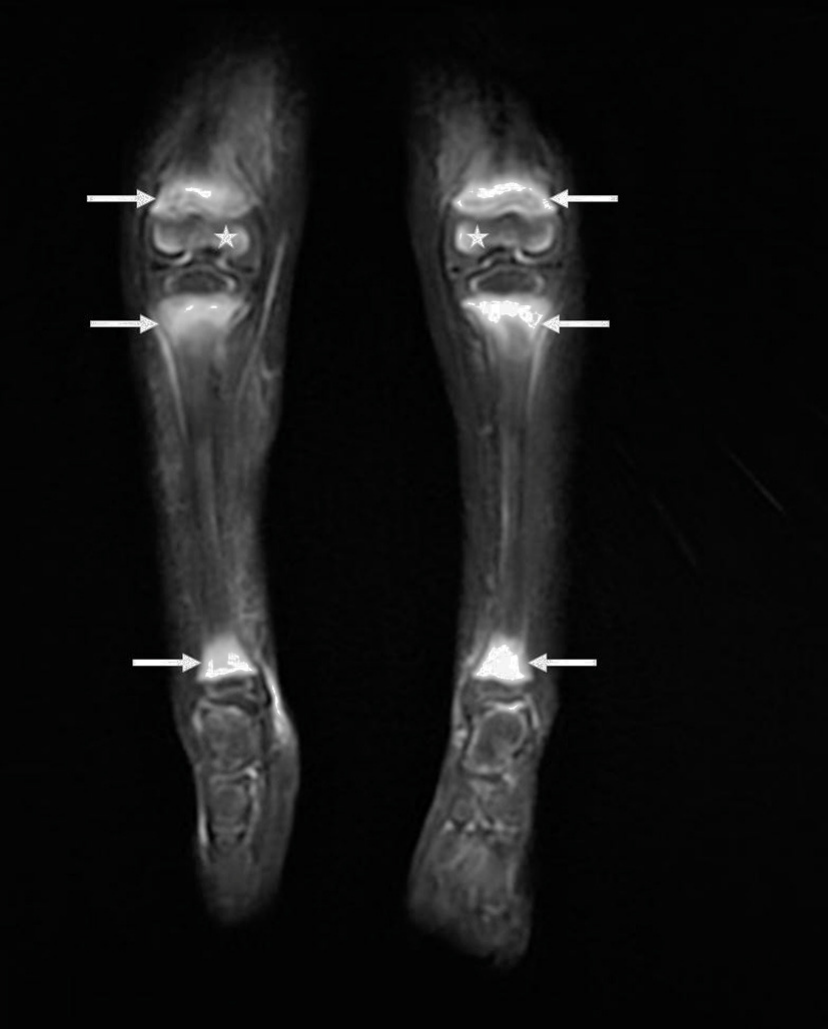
Bilateral lower leg MRI for case 2, a 3.5-year-old girl: coronal fat-suppressed T2-weighted image revealing bilateral bone-marrow edematous changes of the metaphyseal area (white arrows) of the ankles and knees. Edematous changes were also noted in the distal epiphysis of the femur bilaterally (stars). Whole-body MRI shows multiple similar anomalies in the wrists and shoulders.
